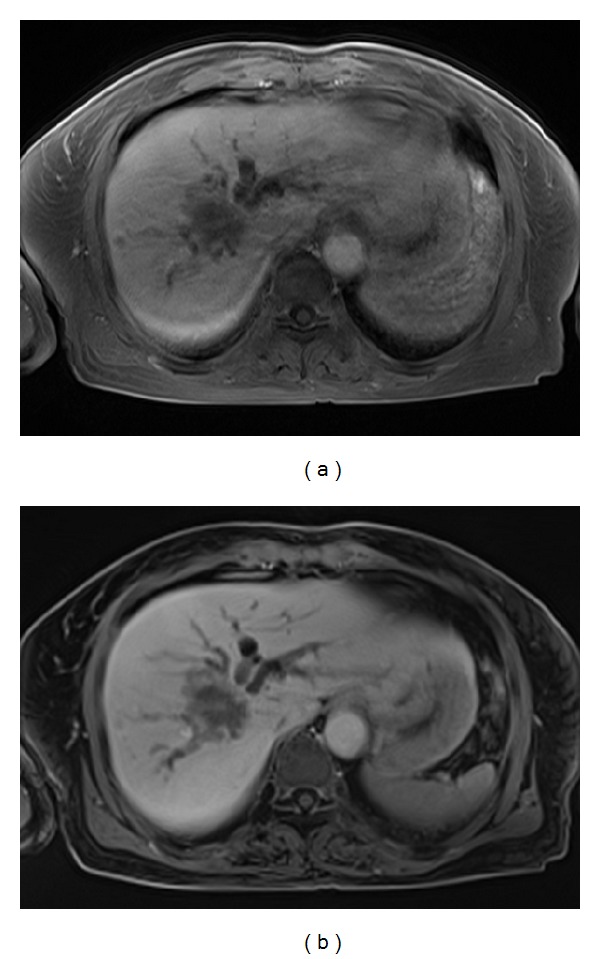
MR images obtained in a 70-year-old woman with an intrahepatic mass-forming cholangiocarcinoma. The T1-weighted 3D conventional breath-hold GRE image (a) shows blurred tumor resolution and dilated intrahepatic bile ducts caused by moderate motion artifacts. The free-breathing T1-weighted 3D radial GRE image (b) shows clear definition of the liver tumor and renders increasing conspicuity of bile-duct invasion. No motion artifacts are present in this image.
